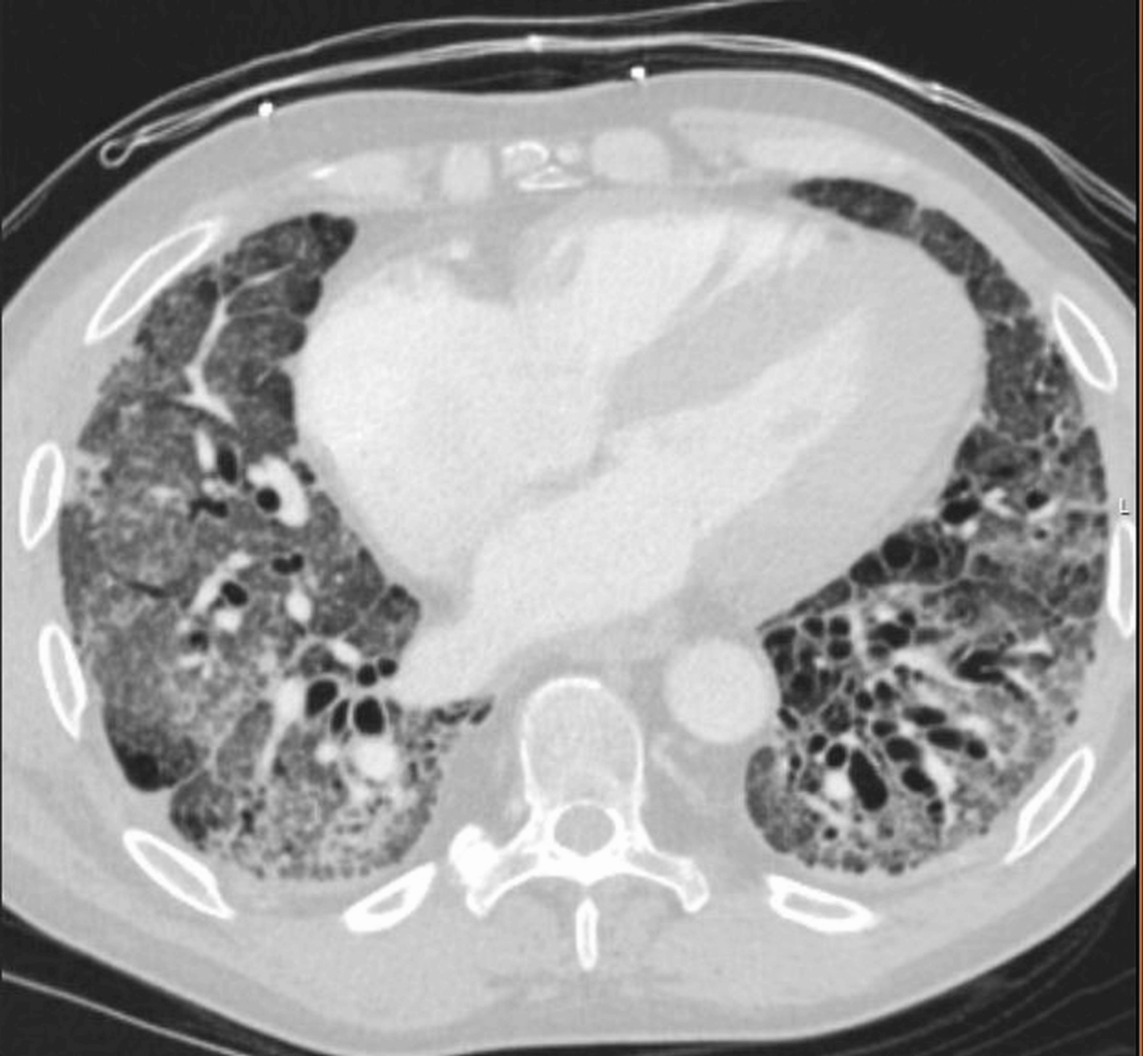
Axial chest CT. High-resolution axial CT image demonstrating bilateral subpleural reticulation, traction bronchiectasis, and fibrotic changes consistent with non-specific interstitial pneumonia. These findings are most prominent in the posterior and basilar regions.
